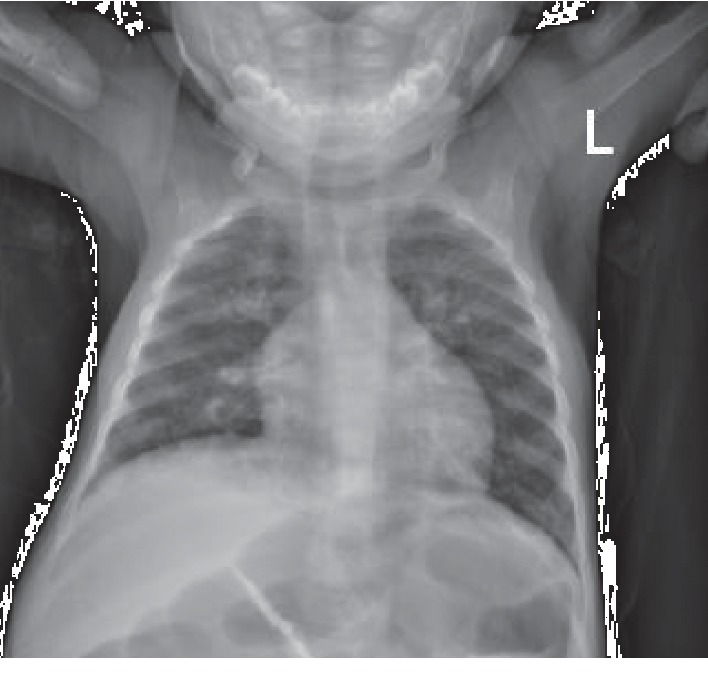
Chest x-ray of the infant.
